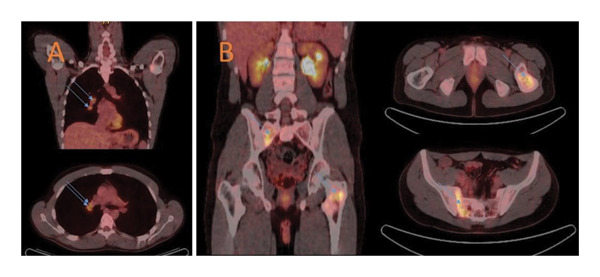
PET/CT scan showing abnormal activity in the sacral region, an abnormal signal in the left greater trochanter and adjacent femoral neck (SUVmax of 4), and two active right hilar lymph nodes measuring 1.5 cm each (SUVmax of 4.7).
